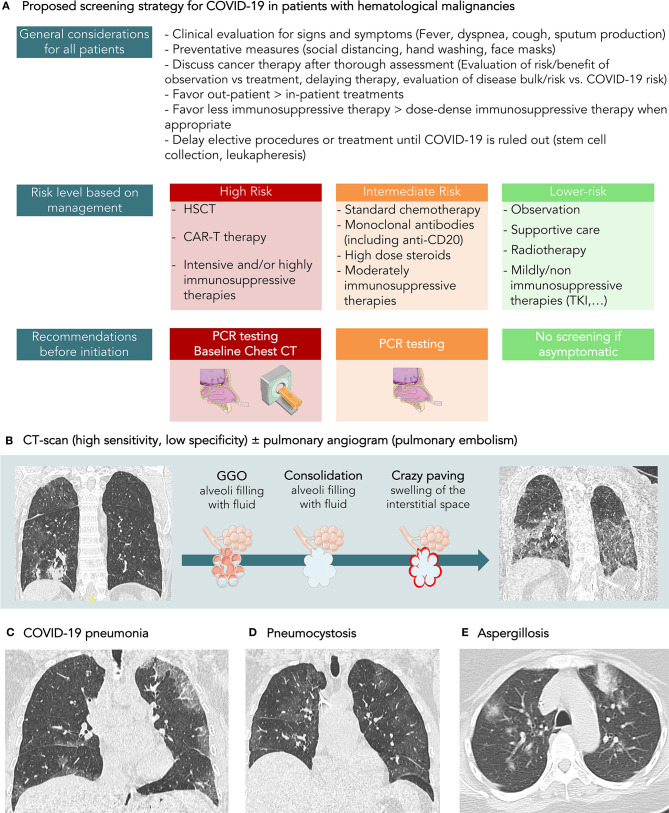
(A) Proposed screening strategy for COVID-19 in patients with hematological malignancies. (B) CT-scan is a sensitive tool, but its specificity for the diagnosis of COVID-19 is around 37%, according to recent meta-analysis. It has not been evaluated in patients with hematological malignancies but could be even lower due to a higher rate of infection caused by immune suppression. (C) COVID-19 pneumonia in a 58-year-old patient with diffuse B lymphoma treated with R ACVBP. Symptoms were cough and fever. SARS-CoV-2 rt-PCR was positive. On CT-scan, the CT score CORADS was 5. The axial CT image showed ordinary COVID-19 pneumonia with multiple regions of subpleural GGO (Ground Glass opacity) with superimposed inter and intralobular septal thickening. (D) Pneumocystis in a 58-year-old patient followed for acute leukemia. The clinical exam revealed a cough and fever. The patient was lymphopenic. CT-scan showed diffused ground-glass opacities. SARS-CoV-2 rt-PCR was negative. The final diagnosis was pneumocystis. (E) Diffuse pulmonary condensation in a 55-year-old patient with AML in febrile aplasia. SARS-CoV-2 rt-PCR was negative. A positive aspergillus antigenemia was confirmed by culture of bronchoalveolar lavage fluid.
